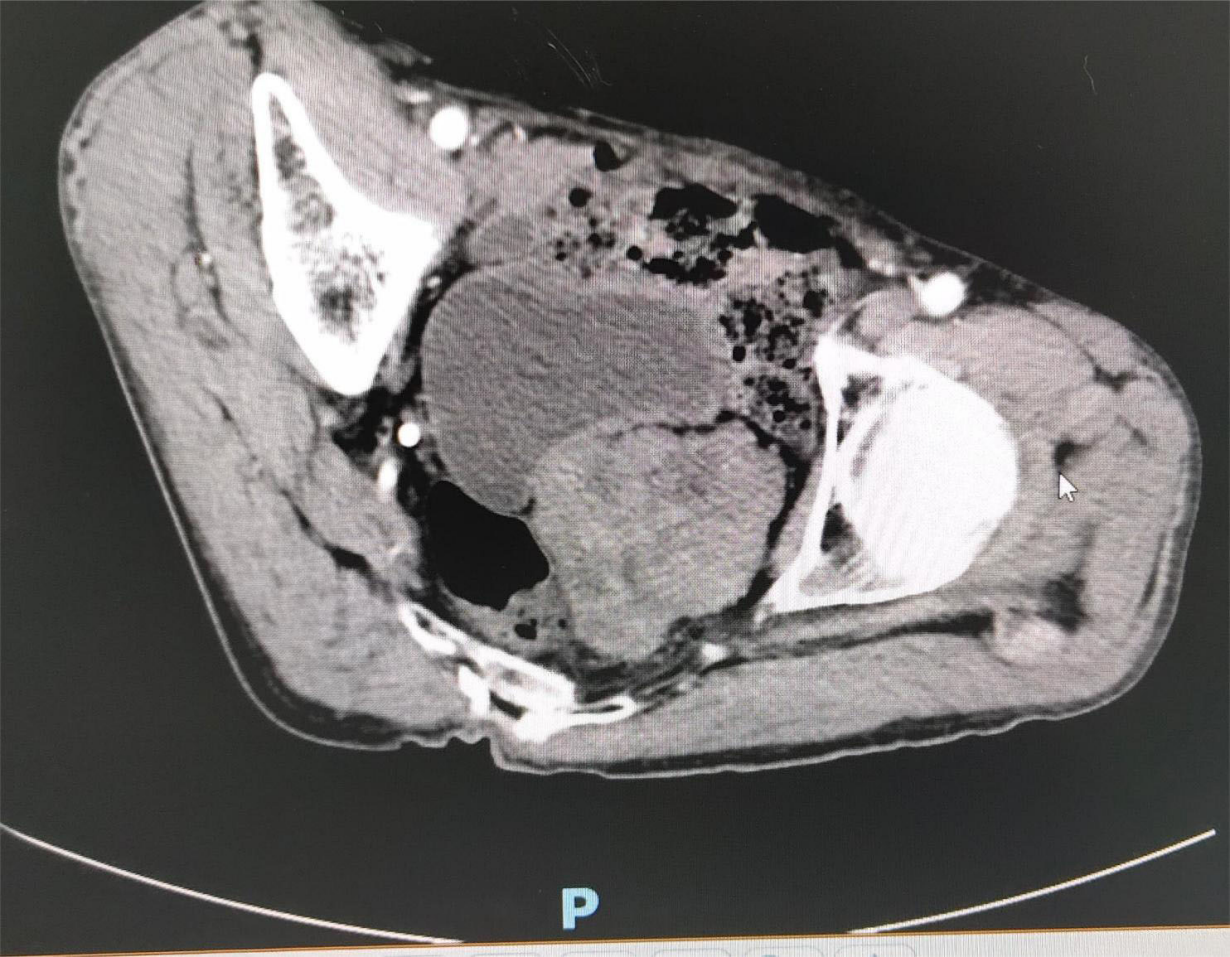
Pelvic CT showing a soft tissue density mass in the left portion of the pelvis, poorly demarcated from the left seminal vesicle glands and prostate, with an unclear border and uneven density.
